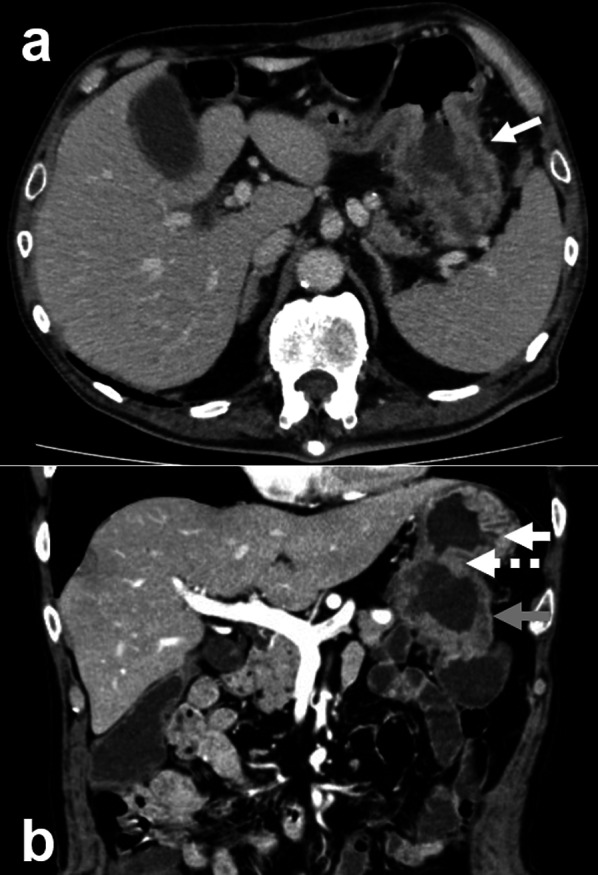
a Computed tomography revealed a tumor in the splenic flexure of the colon, and the tumor had invaded the greater curvature of the stomach and the tail of the pancreas. The white arrows indicate gastrocolic fistula. The spleen was enlarged, and there was suspicion of tumor invasion to the splenic vein. b The tumor was mainly located in the splenic flexure of the colon, and except for the greater curvature where the tumor invaded, there were no obvious abnormalities in the stomach. The white, white dotted, and grey arrows indicate the stomach, gastrocolic fistula, and splenic flexure of the colon, respectively
